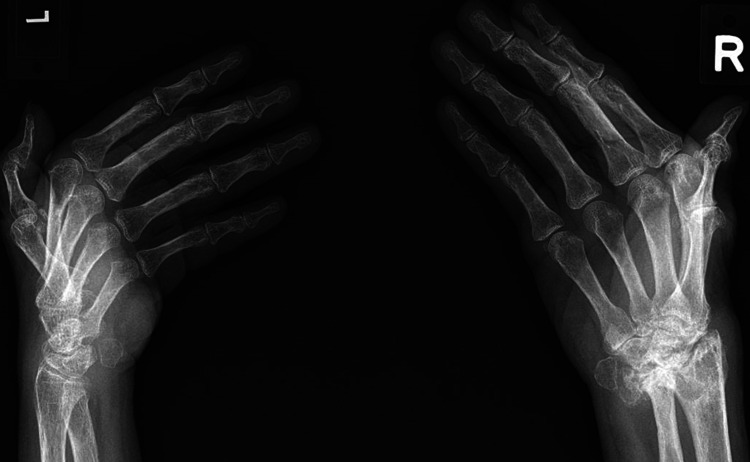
X-ray of the hands, ball catcher’s view
